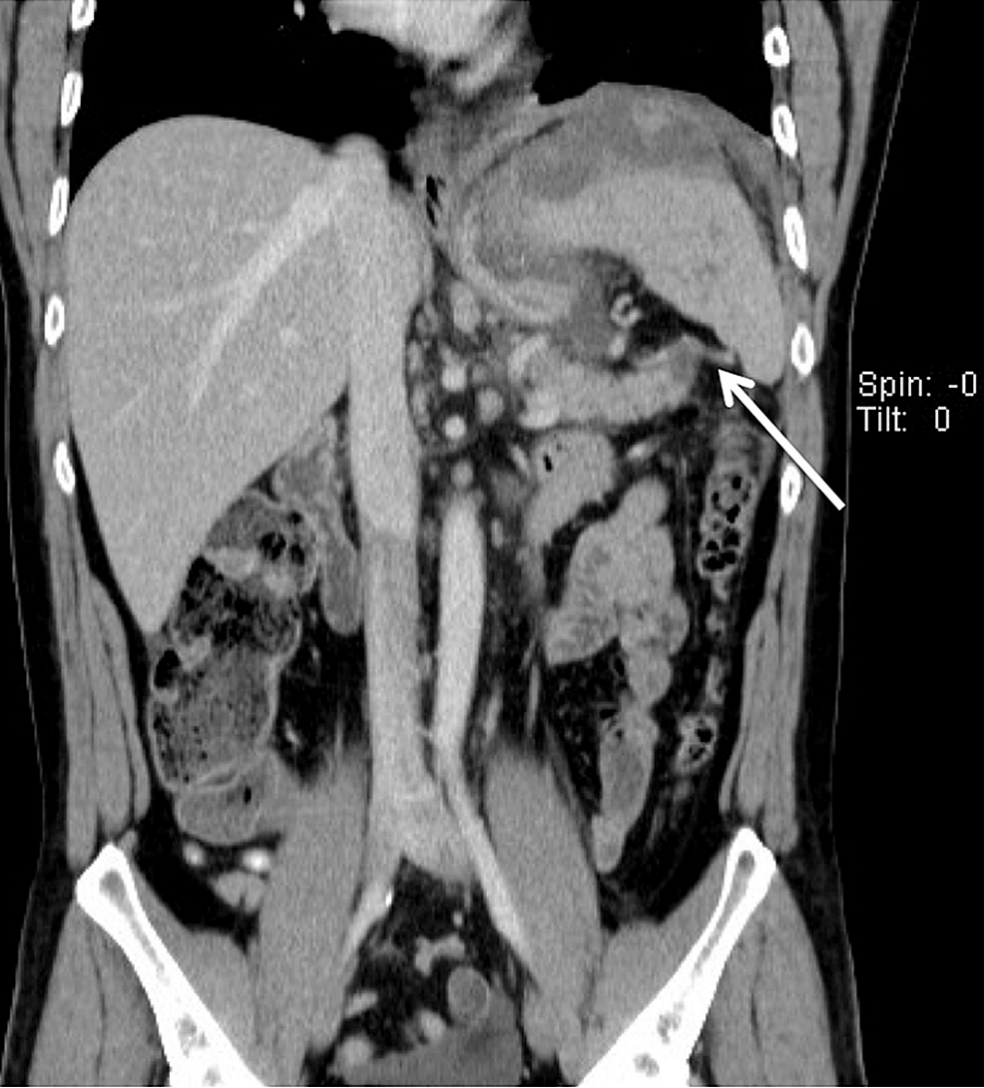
Contrast-enhanced CT scan (coronal plane) 24 hours after admission showing the heterogeneous collection that extends along the subphrenic space, perisplenic region and pancreatic tail.
